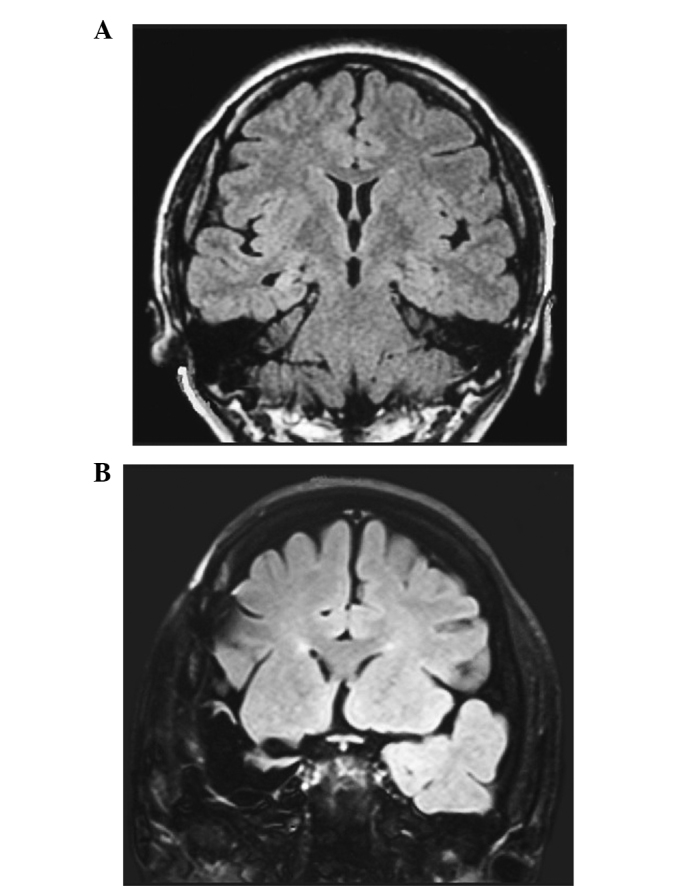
(A) Presurgical fluid-attenuated inversion recovery magnetic resonance imaging (FLAIR MRI) revealed an increased signal in the right mesial temporal structures, suggesting right mesial temporal sclerosis. (B) Postsurgical T1-weighted MRI confirmed the total removal of the right epileptogenic foci.
